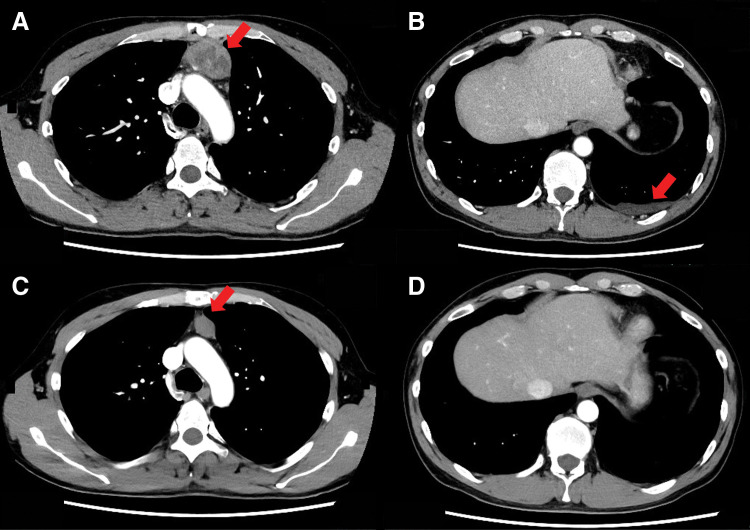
Case 1. Finding of chest computed tomography (CT). (A) Chest CT showed an 87 × 52 × 38 mm multilocular cystic tumor in the anterior mediastinum with contrast enhancement in the walls (arrow). (B) A small amount of pleural effusion was observed on the left side (arrow). (C, D) Chest CT 2 months after the first presentation revealed tumor regression (arrow) and disappearance of the pleural effusion.
